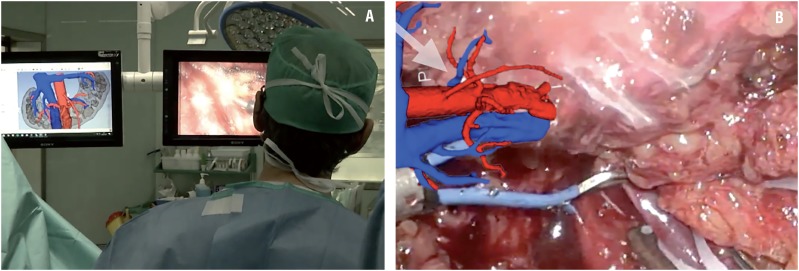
A) During the procedure, CT images and 3D reconstruction were available on the operating theater 2D screens as well as the patient-specific 3D-printed renal model. B) The 3D model faithfully reproduced the real anatomy of the patient and was useful for a better laparoscopic navigation and to confirm the identity of the vessels during the surgery.
